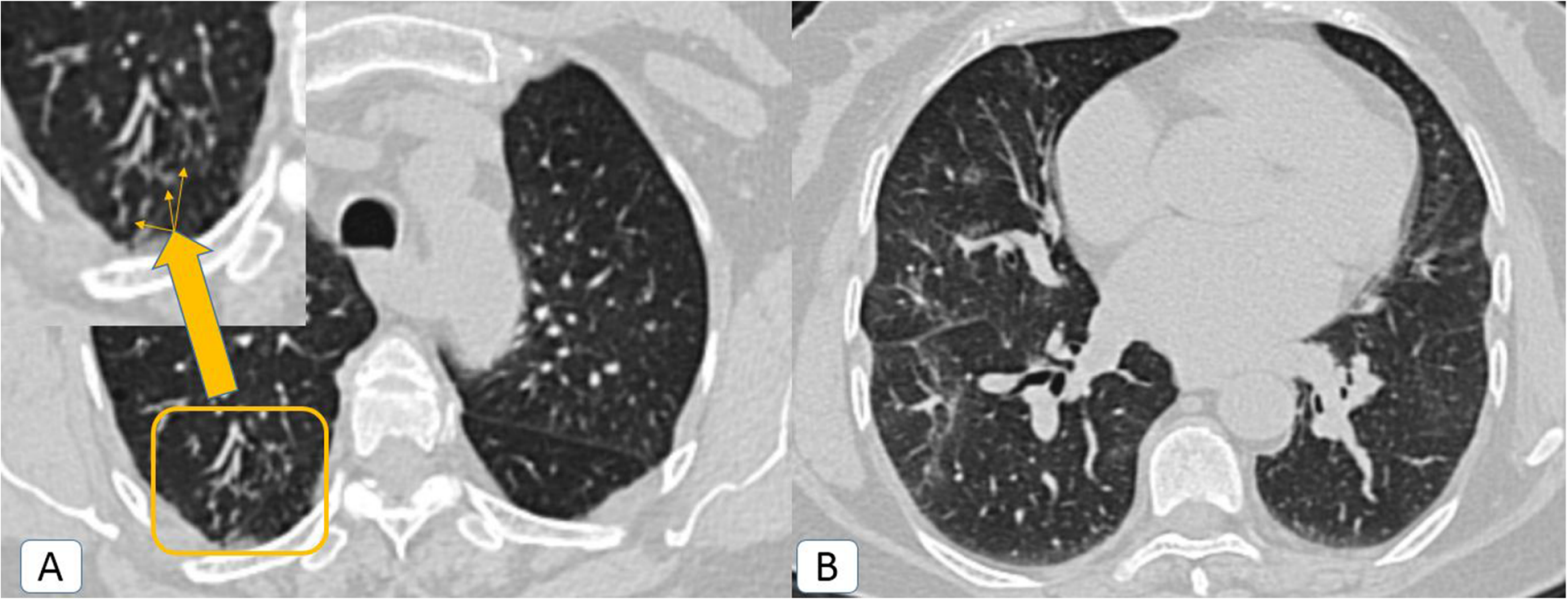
COVID-19 infection atypically presented by tree in bud nodules: a 71-year-old female COVID-19 patient. A Axial chest CT (lung window) showing small tree in bud branching peripheral sub-pleural micro-nodules in the posterior segment of the right upper lobe (yellow square and yellow arrow pointing to zoomed image). B Axial chest CT (lung window) showing bilateral ground-glass patches as well
